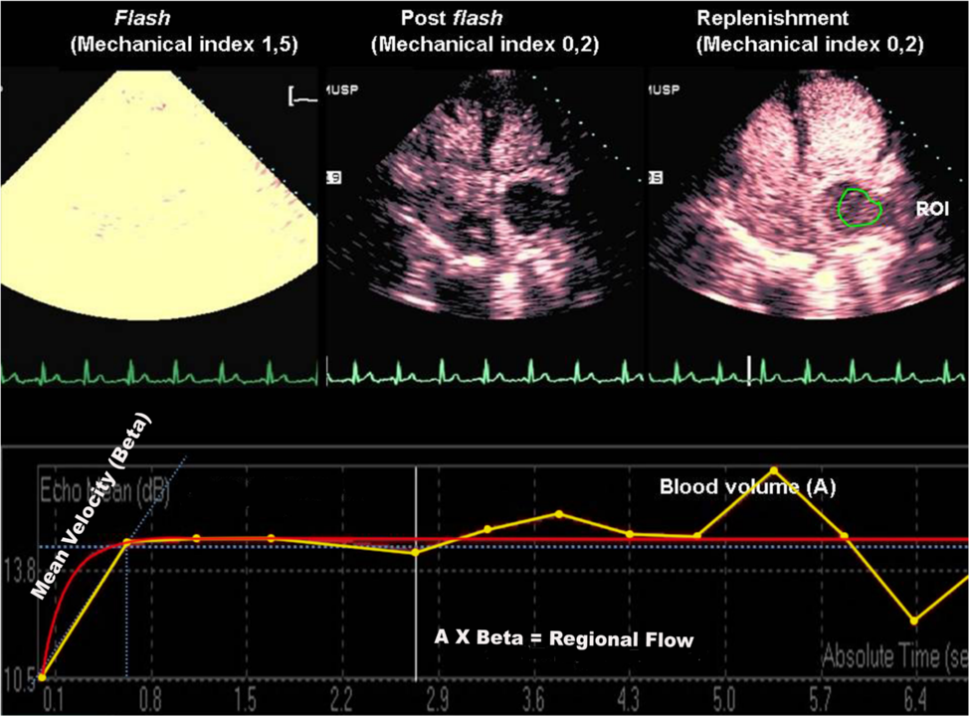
Images from real-time perfusion echocardiography (upper panels) in apical 4-chamber view in a patient with a malignant tumor in the left atrium. Image at left demonstrates the flash impulse high mechanical index followed by post-flash image (middle) and replenishment by contrast (image at right) with region of interest (ROI) manually traced in the tumor. Lower panel shows the time-intensity curve obtained by quantification software with estimation of myocardial blood volume at peak intensity (A), blood flow velocity (*β*) and regional blood flow (A x *β*). Yellow curve was defined by measurements of intensity at each time interval while red curve corresponded to exponential fitted time-acoustic intensity.
